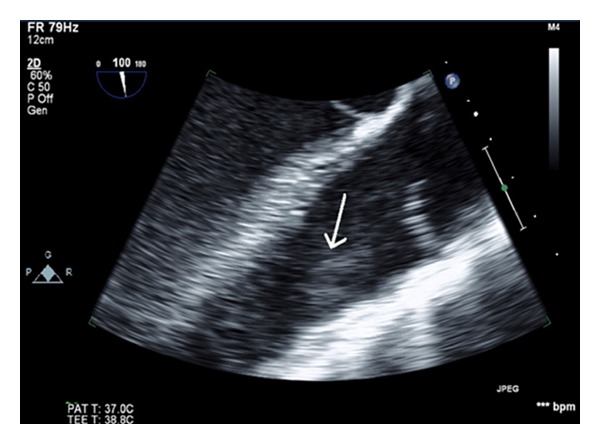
Transesophageal echocardiogram showing a pulsatile mass at the pulmonic valve.
